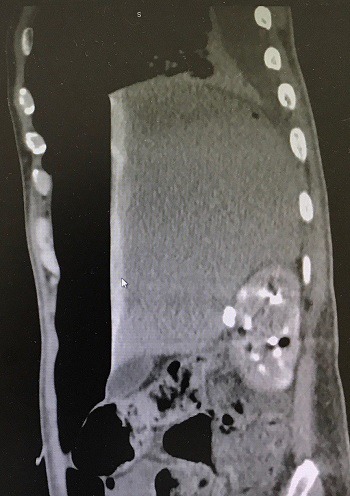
CT Scan was 2 days after the first operation, omental Graham patch operation, showing massive hydro pneumoperitoneum
